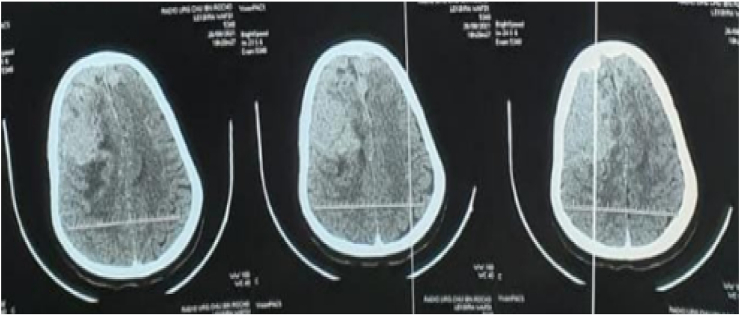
CT-scan was performed objectifying a right frontal extra axial process with extension at the left frontal level intensively taken the contrast medium.
